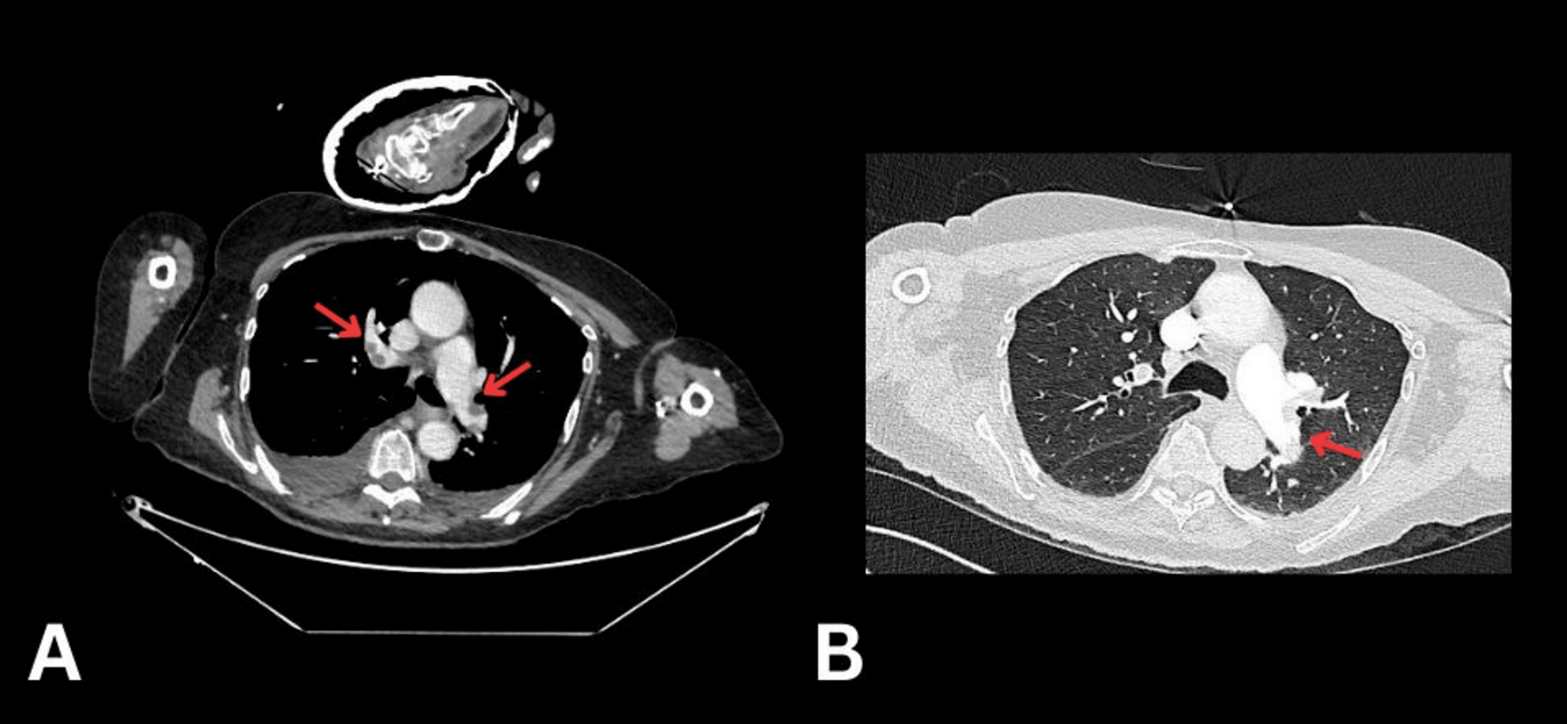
Computed tomography of the chest, abdomen, and pelvis (CT PA). The arrows indicate the presence of thrombus in the pulmonary circulation.
